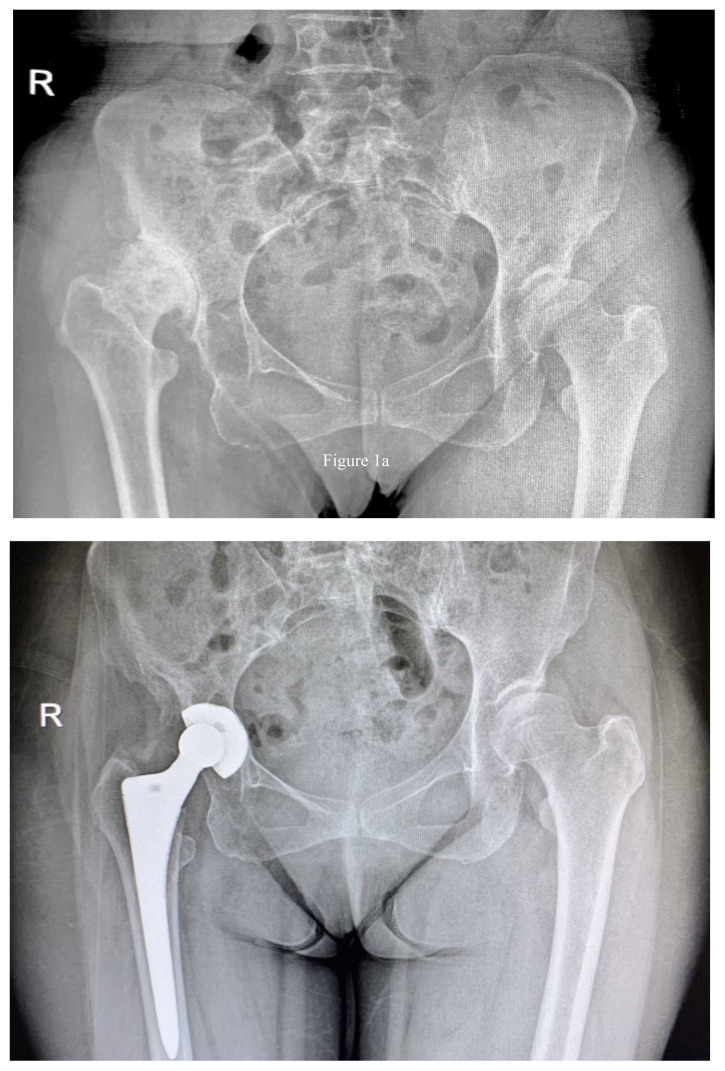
(a) Preoperative X-ray of a Crowe type II hip, (b) postoperative X-ray of THA taken 3.1 years later. The right hip was treated with the press-fit technique.
